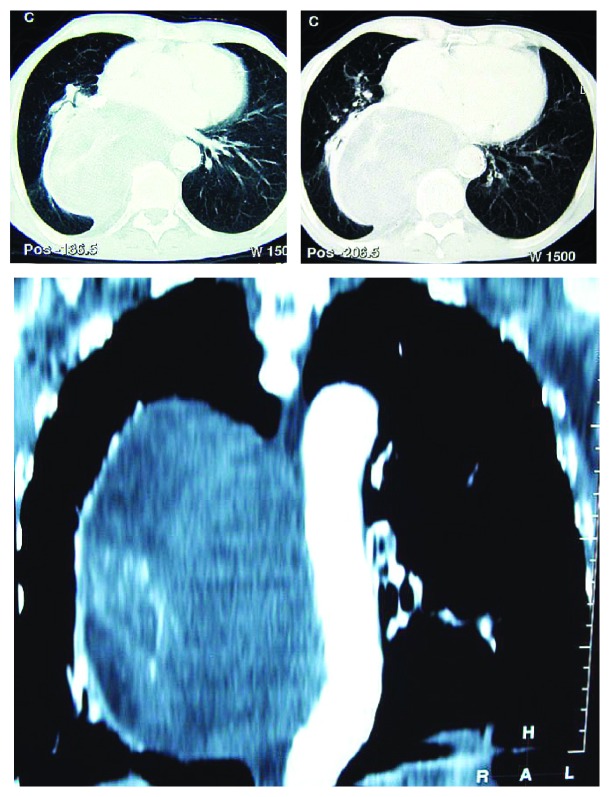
Preoperative CT scan: well-delineated mass with fatty densities and compression on the lung, trachea, right bronchus, and esophagus.
